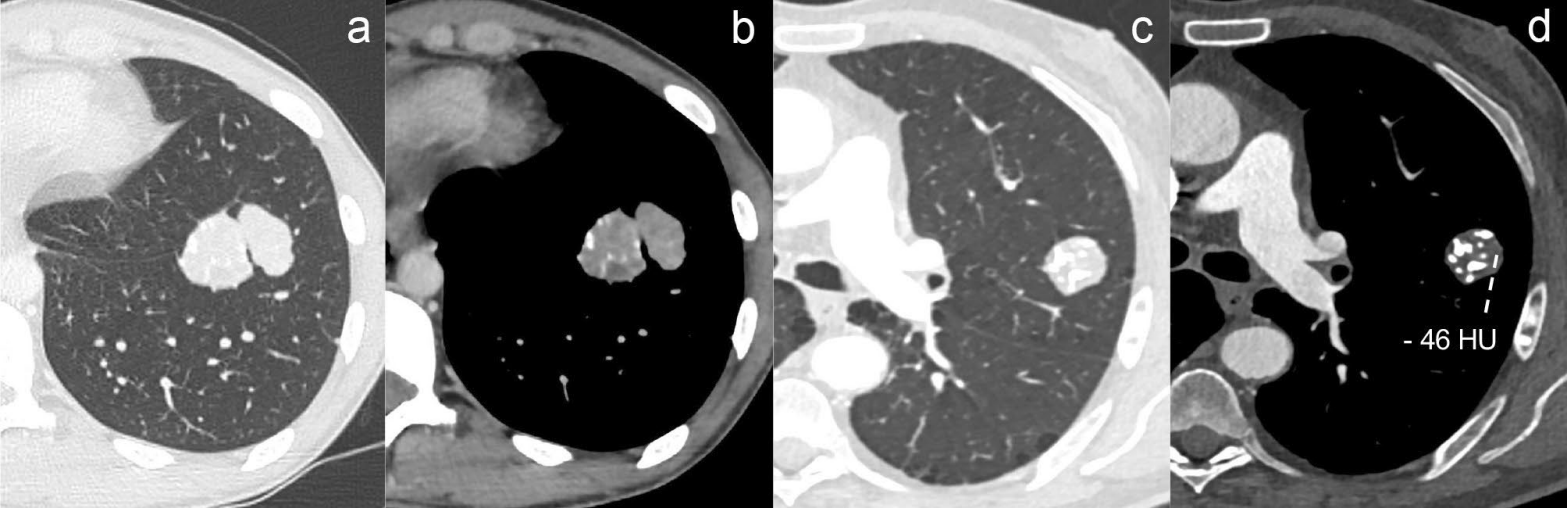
Pulmonary calcified metastases versus pulmonary calcified hamartoma. a, b Pulmonary calcified metastases in a 23 y.o. man affected by osteosarcoma. Contrast-enhanced axial computed tomography (CT) images showing two adjacent solid nodules with inhomogeneous contrast enhancement and eccentric irregular calcifications. c, d Pulmonary calcified hamartoma in a 73 y.o. man with a history of pancreatic adenocarcinoma, treated with pancreatectomy and adjuvant radio-chemotherapy. Axial CT images depict a nodule showing central dense calcifications with popcorn-like appearance; note the associated fat tissue density (-46 HU) within the nodule
